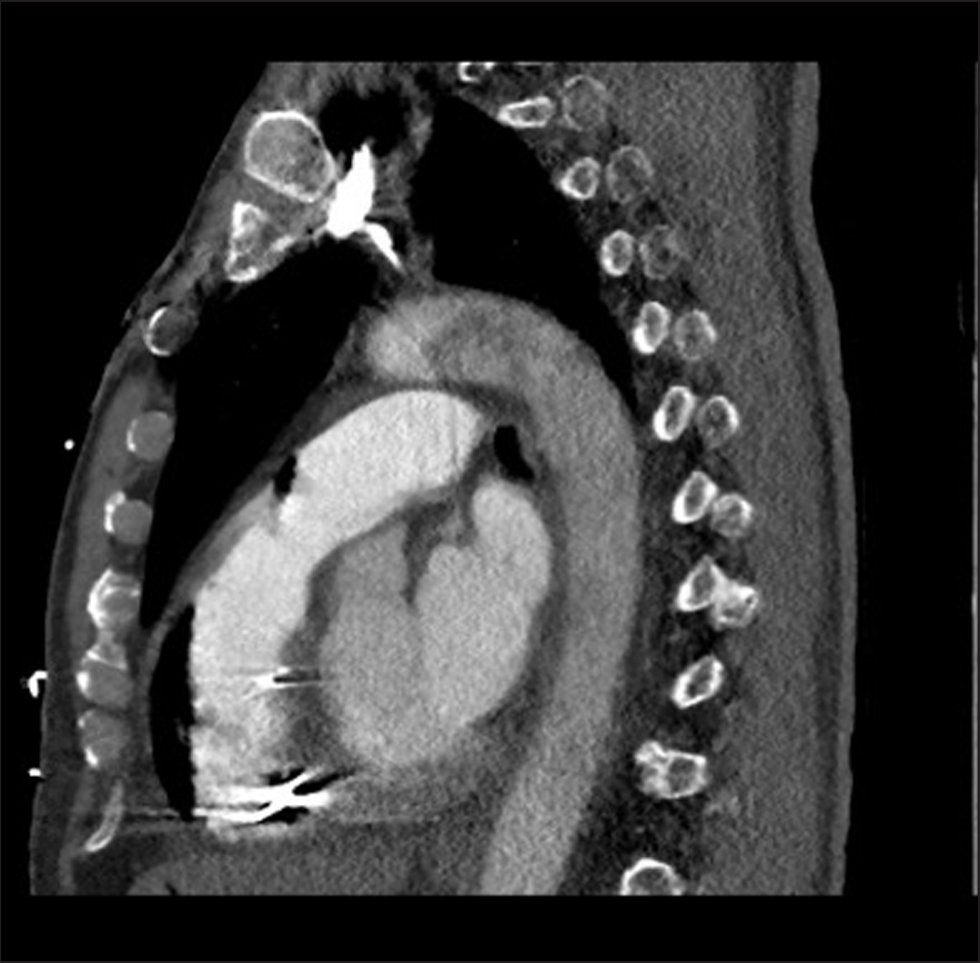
Computed tomography sagittal view demonstrating right ventricular air embolism with extension into the pulmonary trunk.
